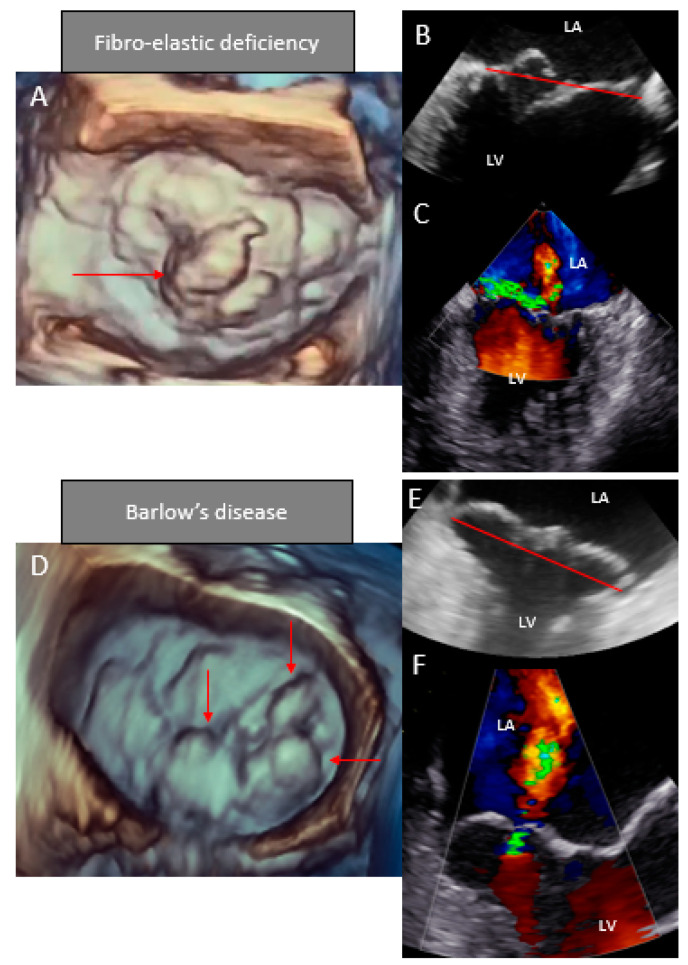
Two-dimensional (color) and three-dimensional transesophageal echocardiographic differences between fibroelastic deficiency and Barlow’s disease. Transesophageal echocardiography including 3D images for a patient with fibroelastic deficiency (A–C) and a patient with Barlow’s disease (D–F). Panels A and D display the 3D transesophageal images, showing the detailed anatomy of the valve with a single prolapsing segment in panel A and a multiscallop, bileaflet prolapse in panel D (red arrows). Panels B and E show the 2D view with the mitral valve annulus plane (red line); the patient with fibroelastic deficiency presented a single segment prolapse and the patient with Barlow’s disease a bileaflet prolapse. Panels C and F show the color-Doppler images with mitral regurgitation. LA = left atrium and LV = left ventricle.
